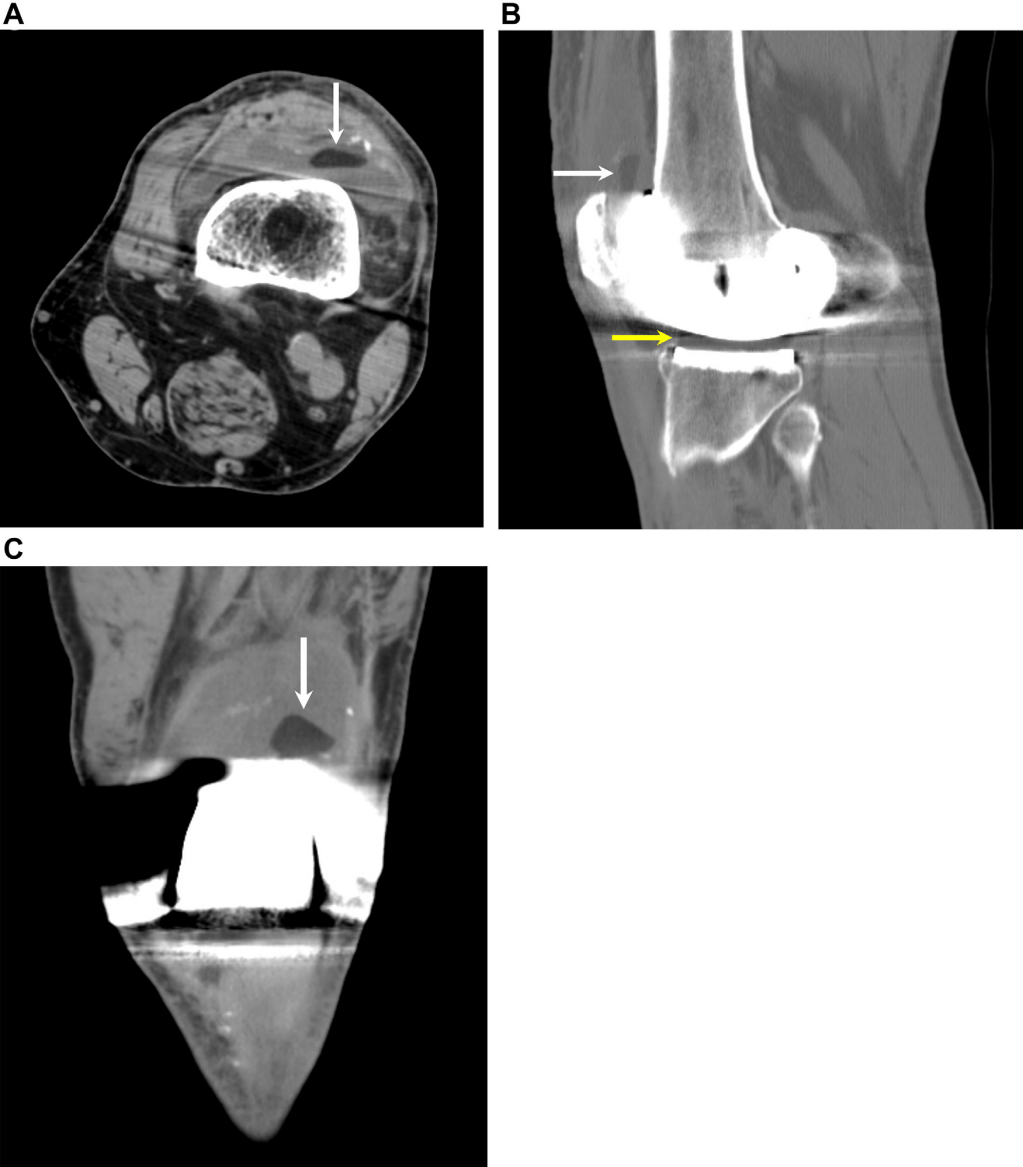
(A), (B), and (C). Axial, sagittal, and coronal CT images show a hypodense structure (white arrow) in the patellofemoral compartment of the left knee joint. The density of this structure is similar to the polyethylene liner (yellow arrow) of the knee arthroplasty.
